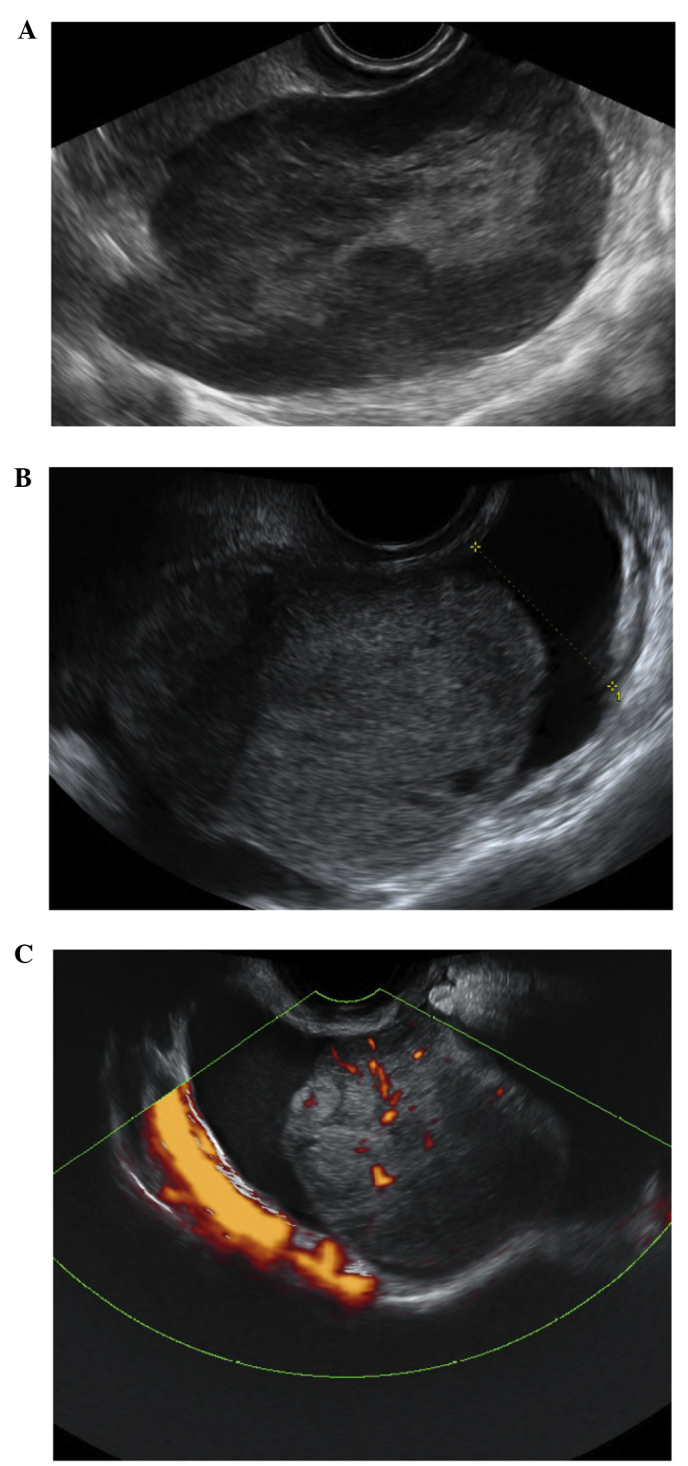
Metastatic cancers to the ovary appear as solid tumors. (A) Lymphoma. (B) Gastric adenocarcinoma. (C) Gastric adenocarcinoma with metastasis to the ovary with using power Doppler 2D image.
